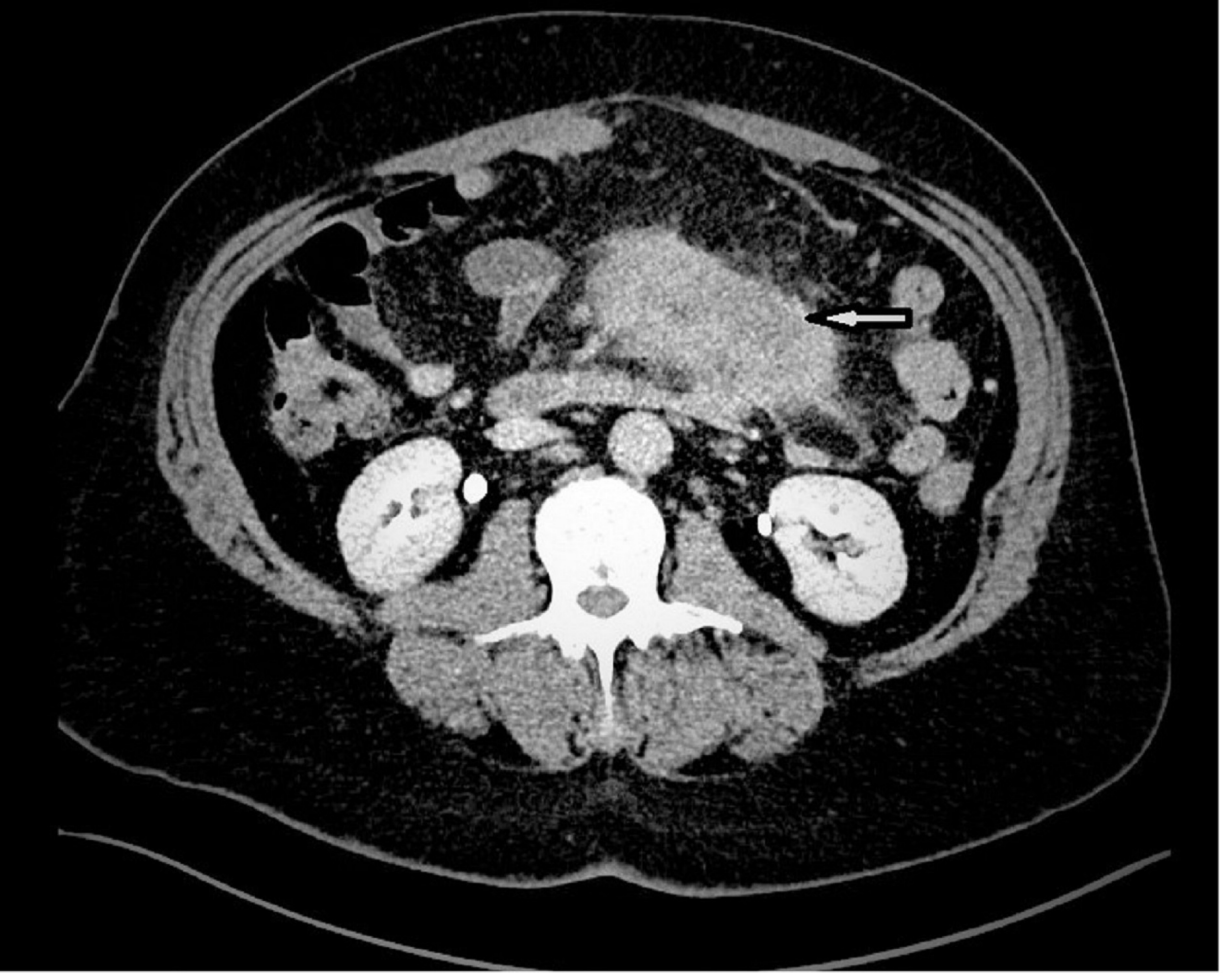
Computed tomography of the abdomen with intravenous contrast showing a large 8 cm mesenteric mass (white arrow) with surrounding stranding and poorly defined borders
